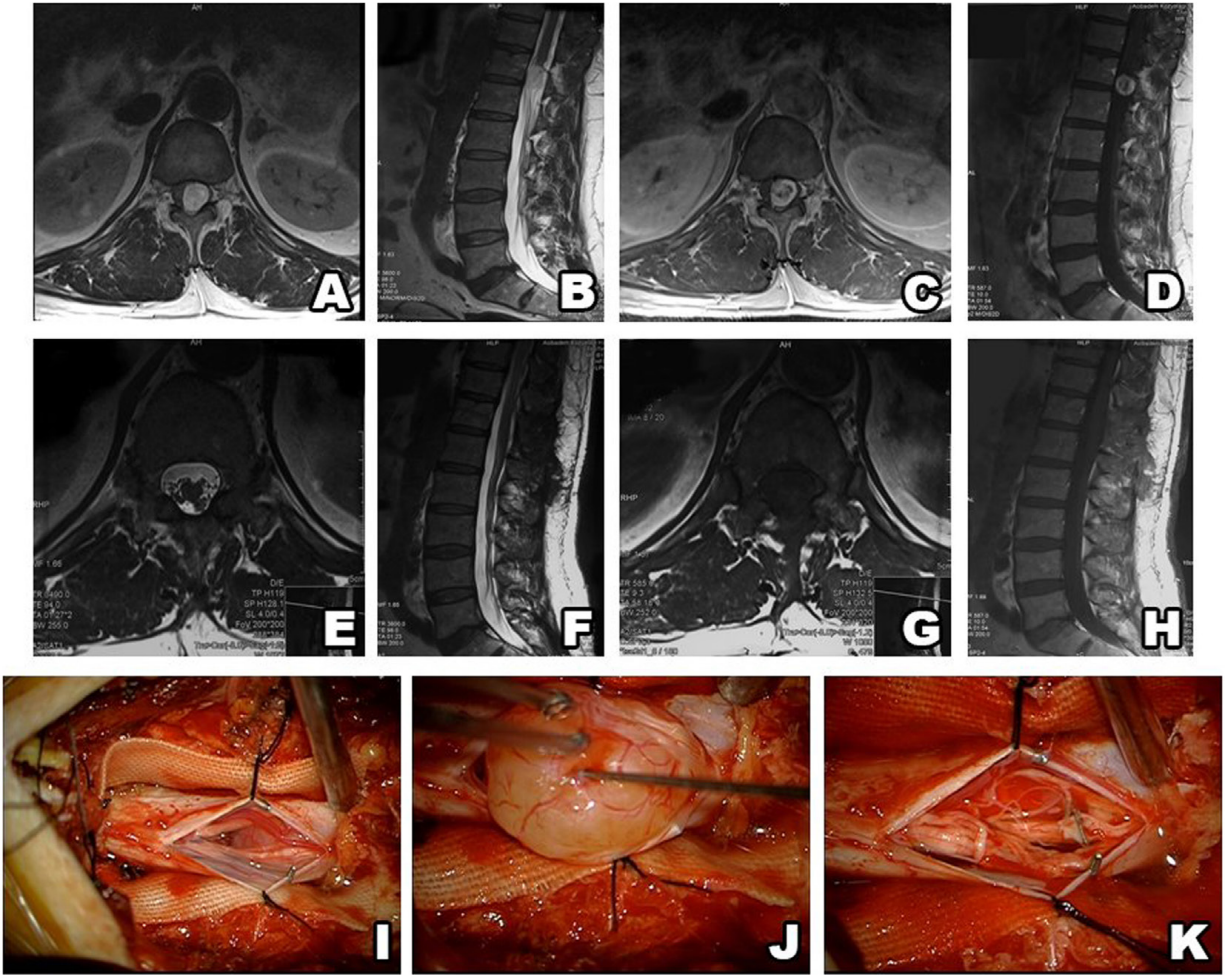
Type I schwannoma (intradural localization). MRI shows a heterogeneous, contrast-enhanced intradural tumor (A–D). Postsurgery MRI confirms gross total resection, without hematoma or recurrence (E–H). Intraoperative photos before and after tumor resection (I–K).
